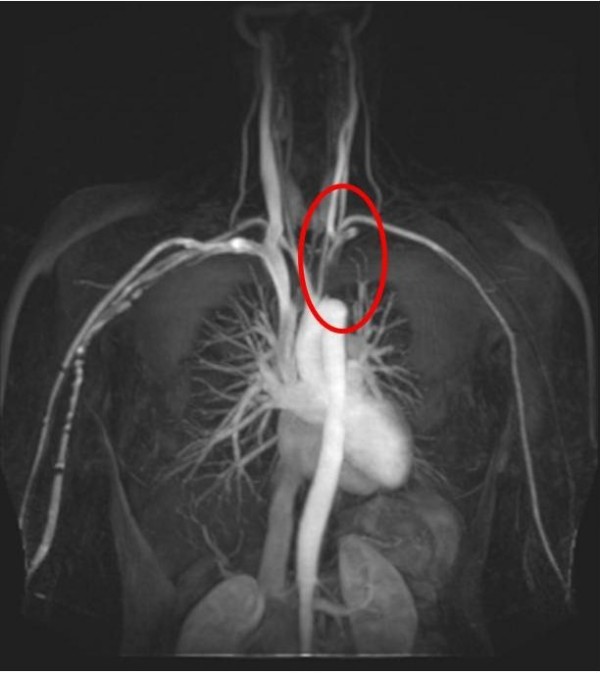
MRI showing complete occlusion of proximal 4 cm of left subclavian artery with retrograde filling via the left vertebral.
